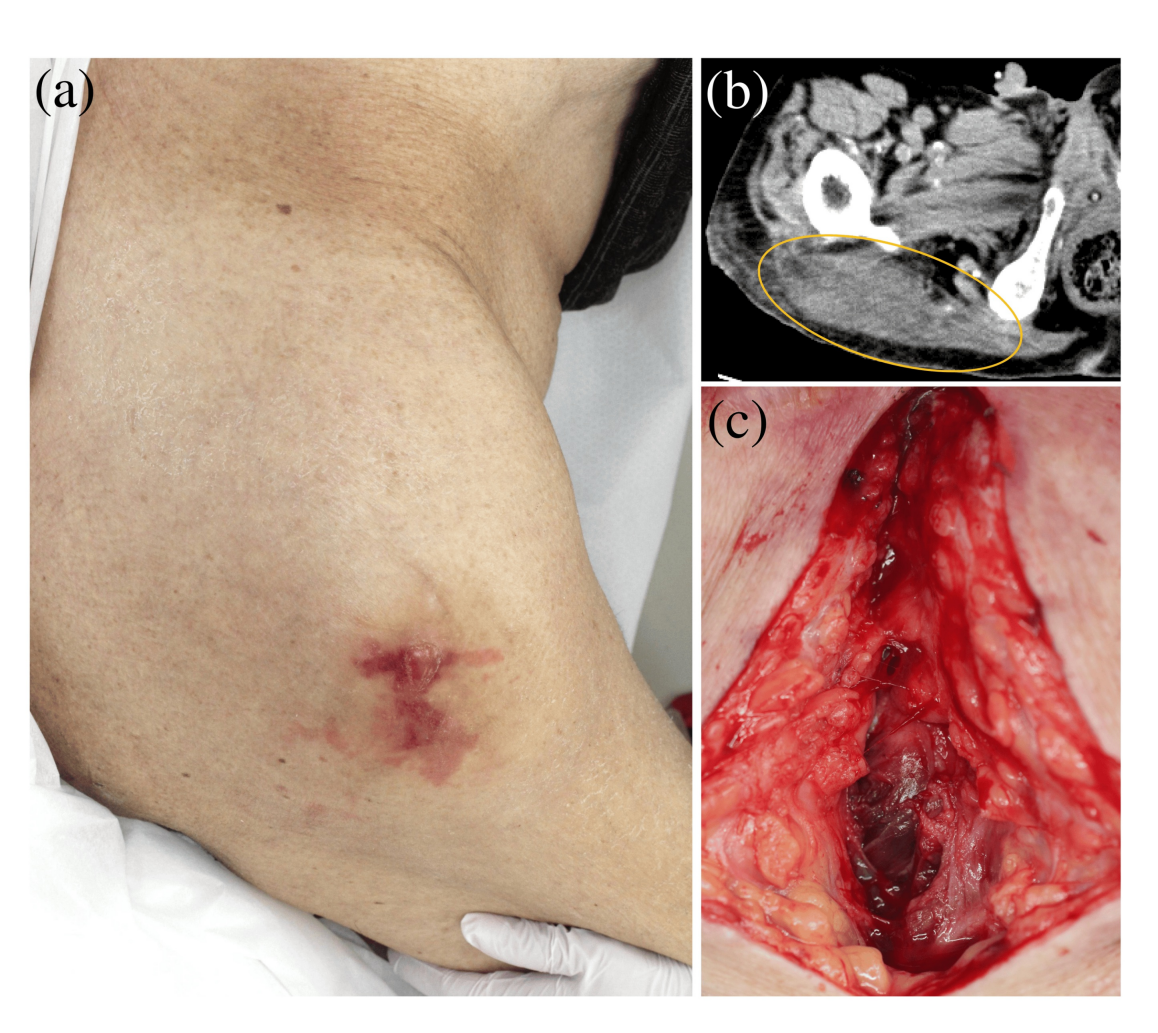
Cutaneous manifestations and CT findings (a) A warm and painful swelling with irregularly shaped erythema and a blister on the right lateral buttock. (b) Swelling of the right gluteus maximus without abscess on CT images (yellow circle). (c) Reddish-brown gluteus maximus without soft tissue necrosis or abscesses. CT: computed tomographic
